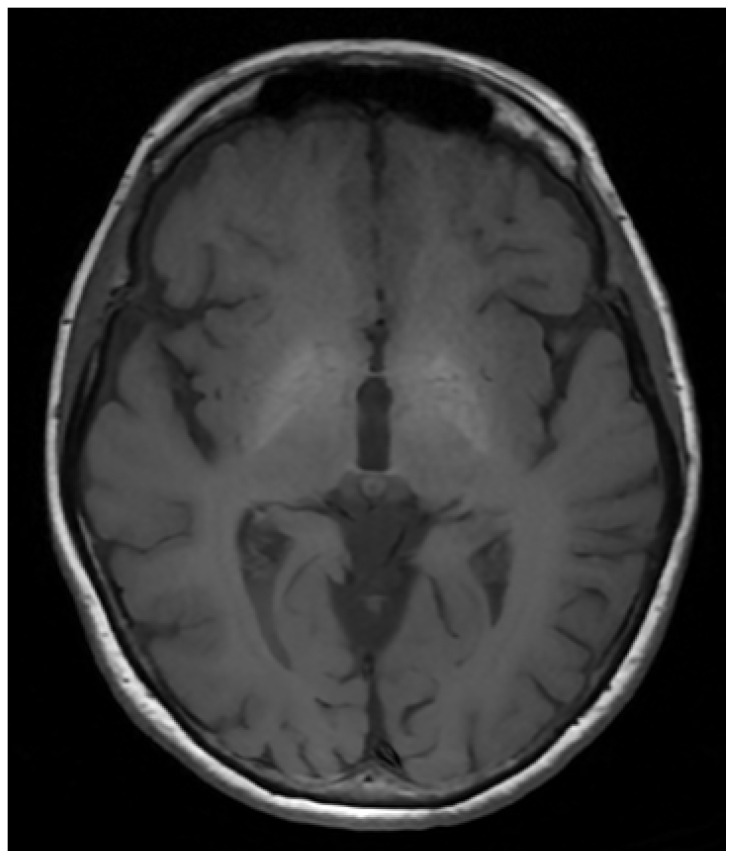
Brain MRI pathology in acquired hepatocerebral degeneration—symmetrical hyperintense signal in T1 sequence in globus pallidus (authors’ material from the Department of Neurology).
